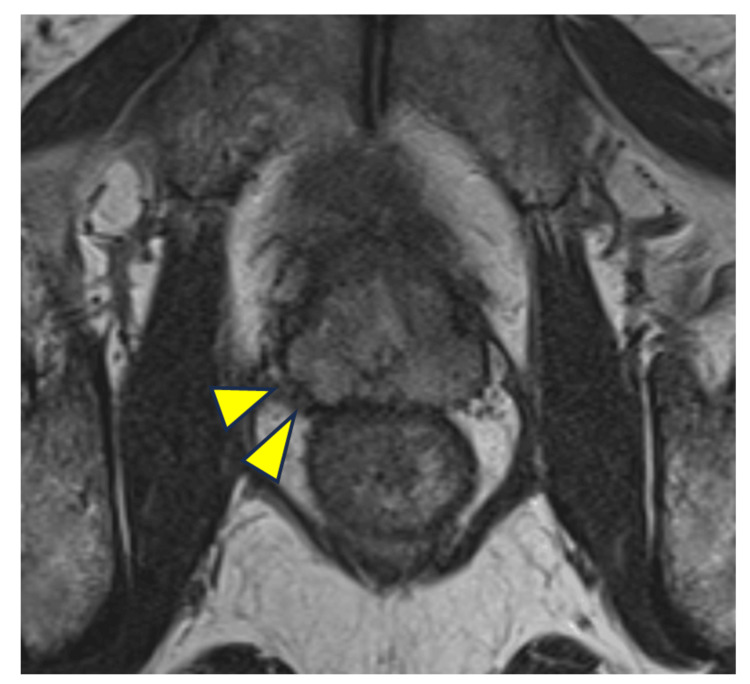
Prostate on MRI after conservative treatment Prostatic and bilateral seminal vesicle swellings improved and fluid collection almost disappeared (arrowheads).
